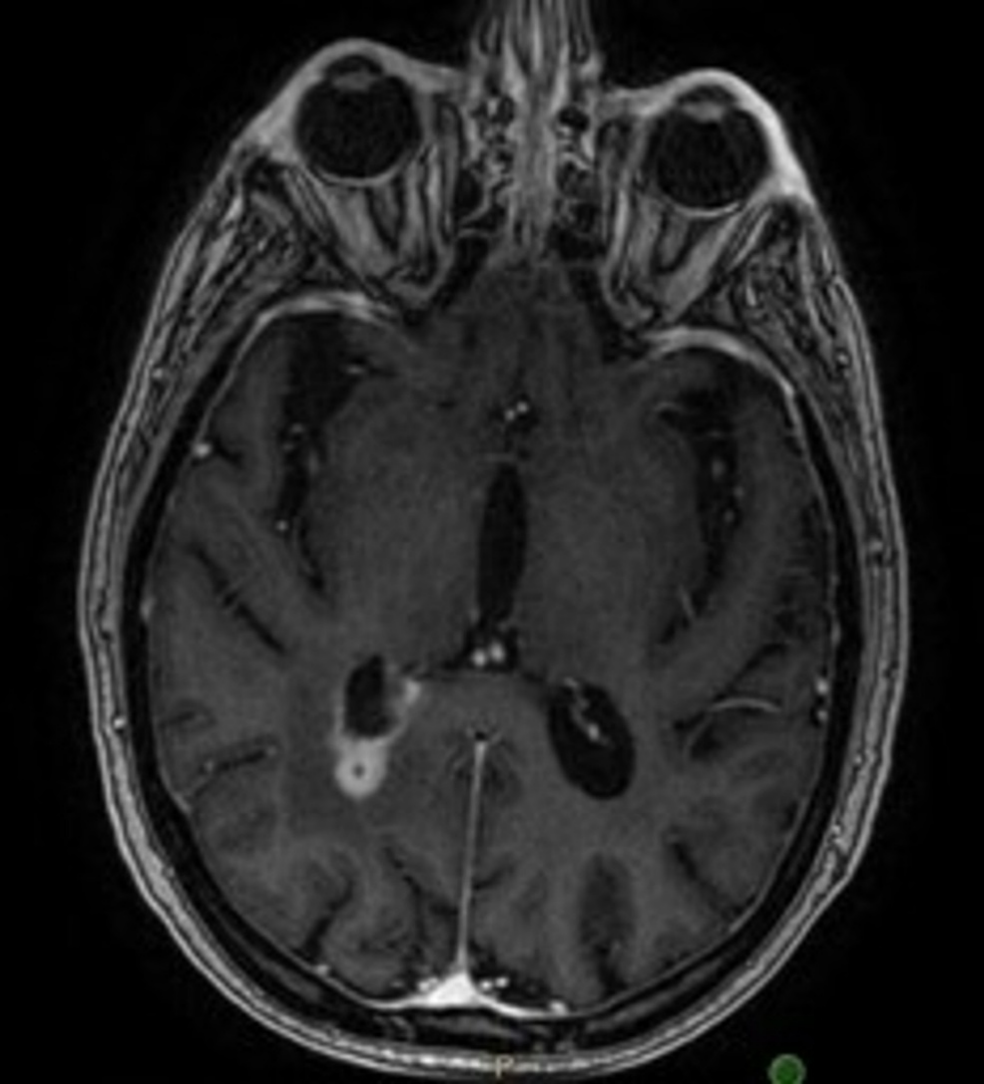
MRI brain with contrast, T1-weighted image showing 1.1 cm right parieto-occipital adjacent to the atrium of the right lateral ventricle
